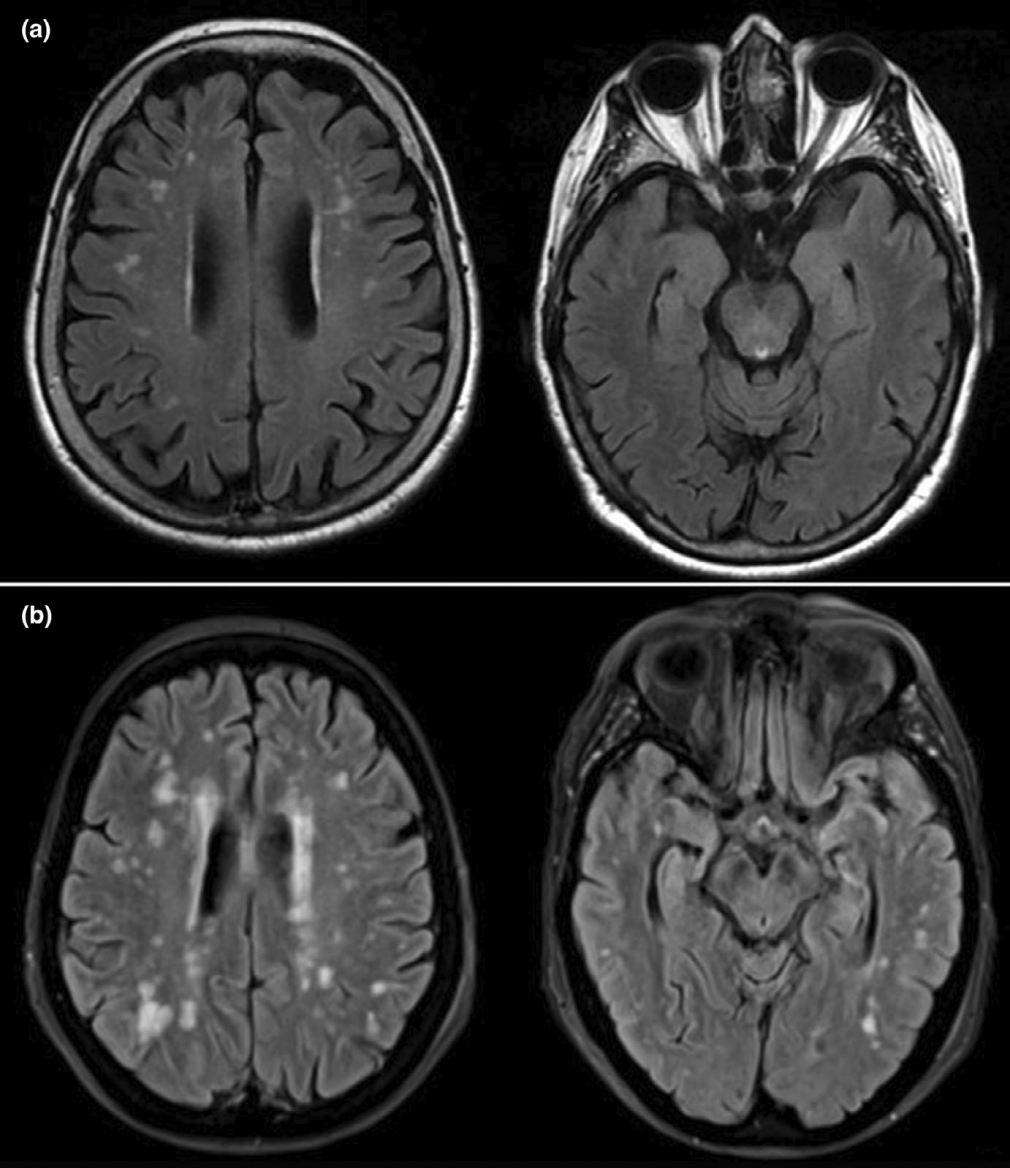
Axial T2 FLAIR MRI images demonstrating hyperintense lesions consistent with gliosis (a) in the right frontal and left temporal lobes; (b) in the frontal lobes.
